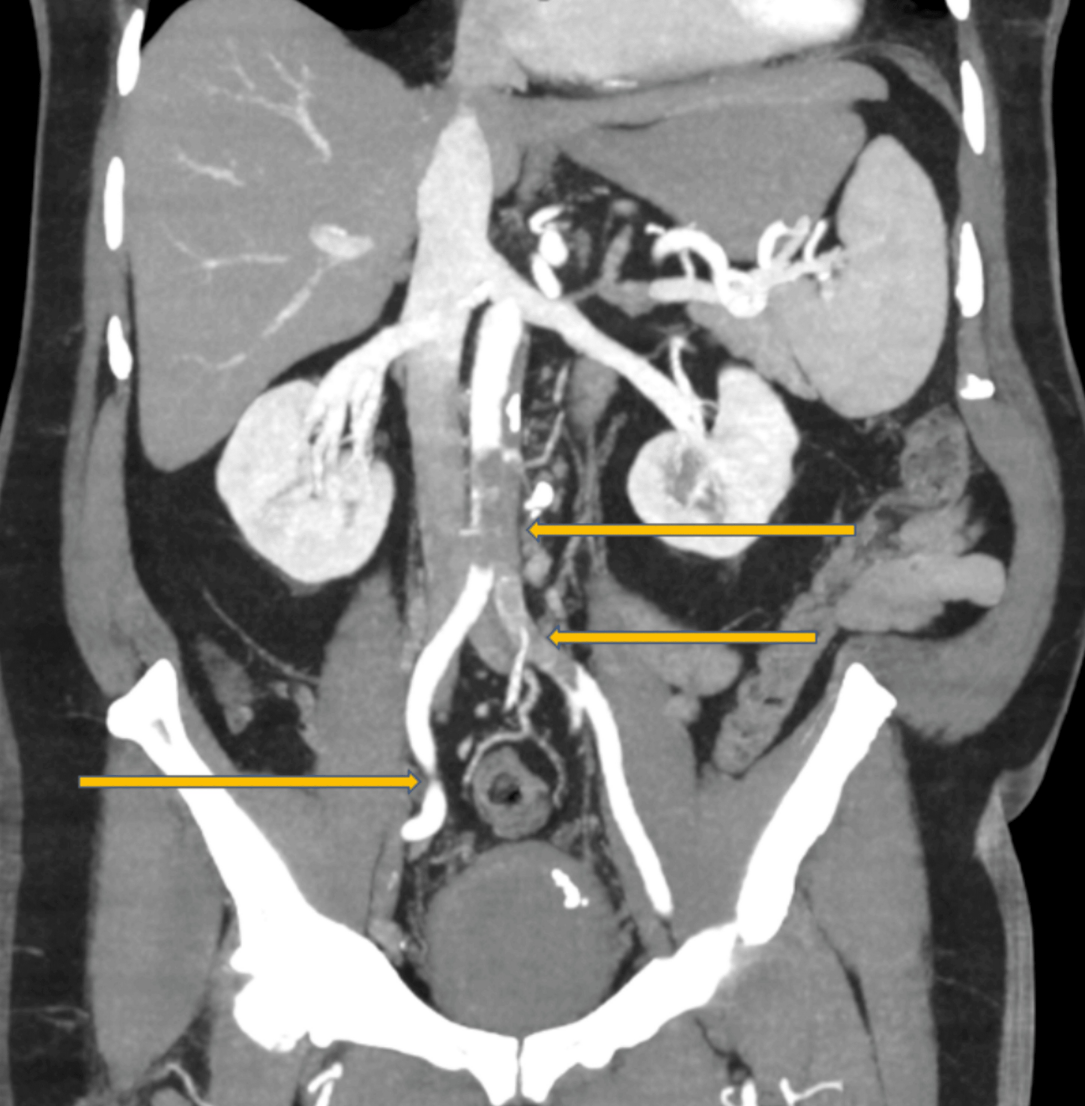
Coronal CT angiography of the abdominal aorta and iliac arteries demonstrating extensive intraluminal thrombus (yellow arrows) extending from the infrarenal abdominal aorta into the bilateral common iliac arteries. The thrombus causes near-total luminal occlusion with markedly reduced distal contrast opacification, consistent with acute aorto-iliac thrombosis. CT: computed tomography
